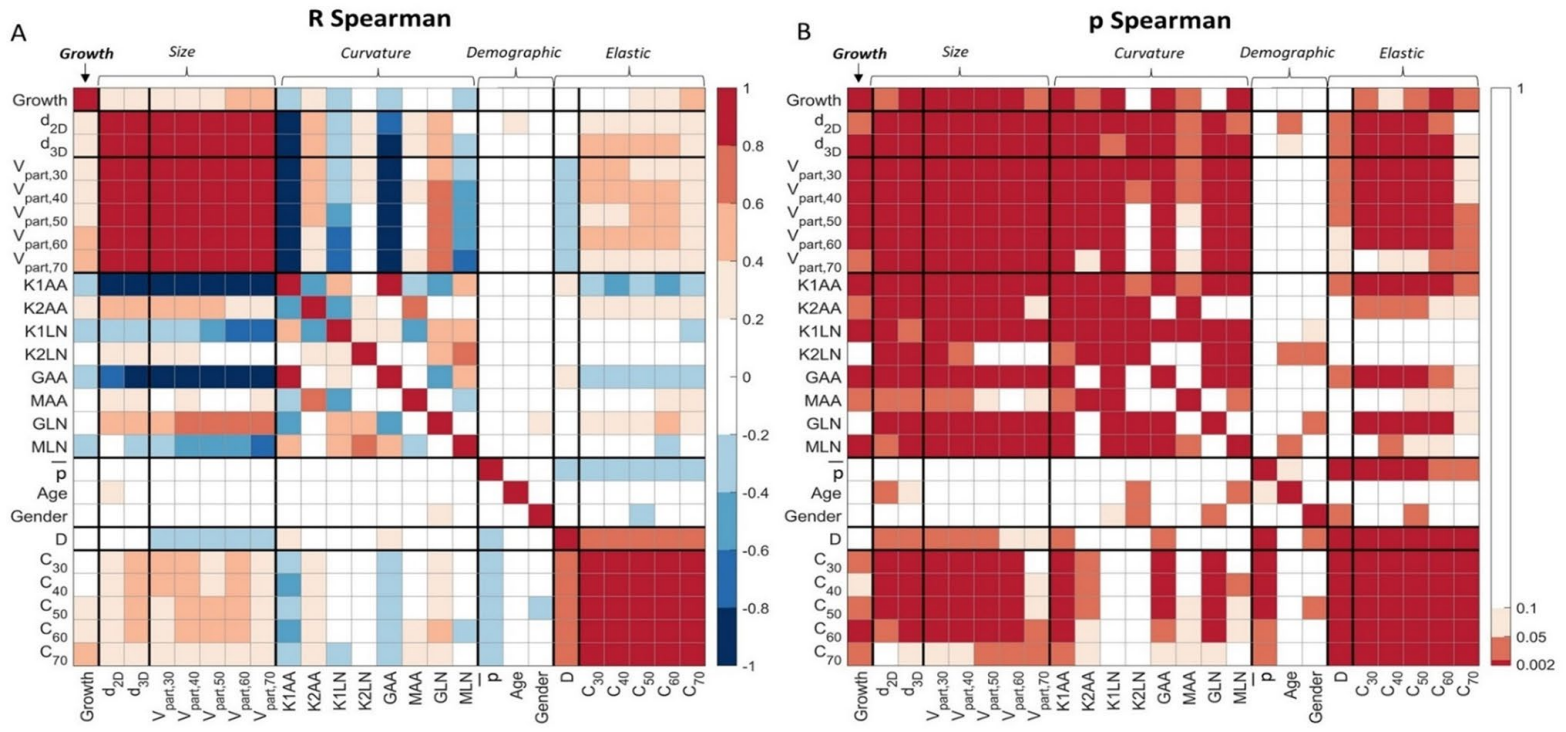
Spearman’s R (A) and correlation p-value (B) for all tested variables. In this, d_2D_ is the diameter from 2D ultrasound, d_3D_ the diameter perpendicular to the centerline from 3D ultrasound, V_part,L_ the volume in a L mm long region around maximal diameter. Furthermore, the area-averages [X]AA and L2-norm [X]LN of the first and second principal curvatures K1 and K2, and of the mean and Gaussian curvatures K and G are shown. p̅ is the mean arterial pressure, D the distensibility, and C_L_ is the compliance in a L mm long region around d_3D_
